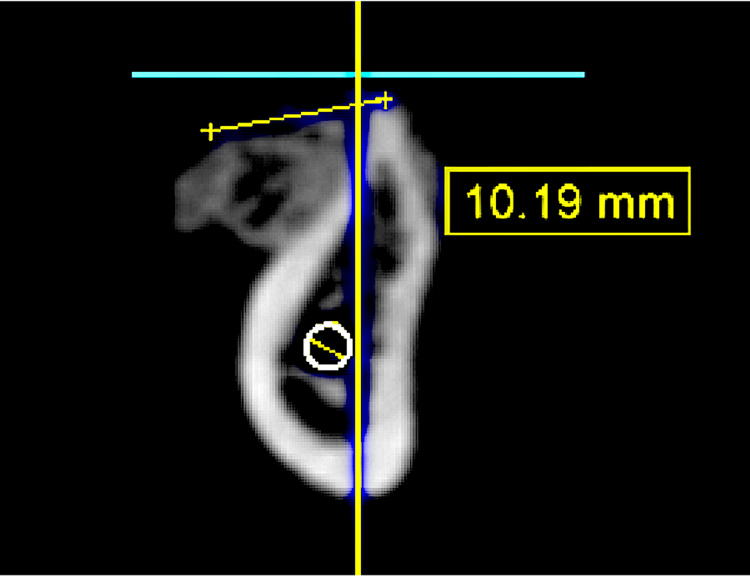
Cone beam CT cross-section to show the grafted site after six months of the surgery.
